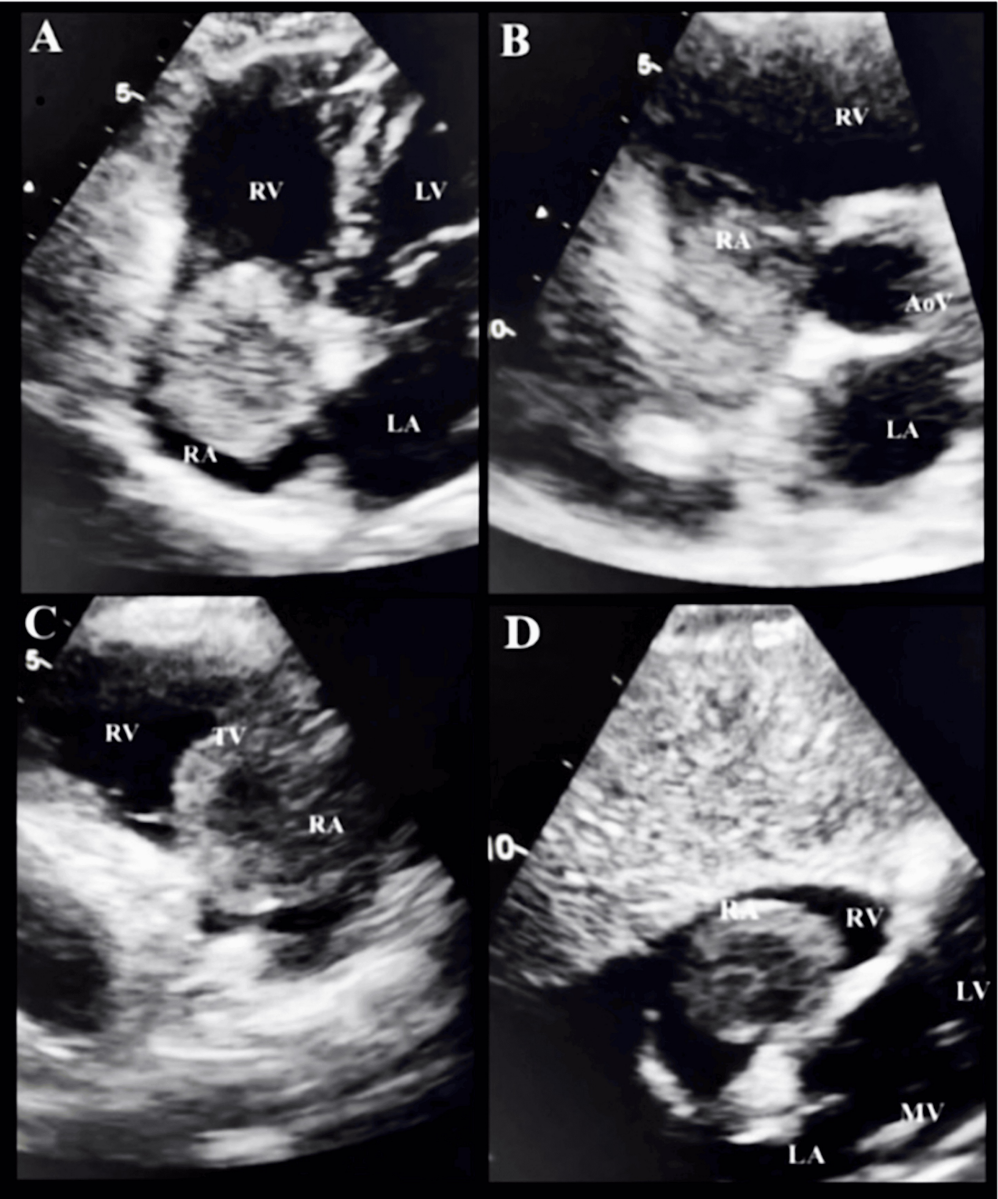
Two-dimensional transthoracic echocardiogram (TTE). (A) Apical view of four chambers, (B) short-axis view, (C) parasternal right atrial view, (D) subcostal view.
